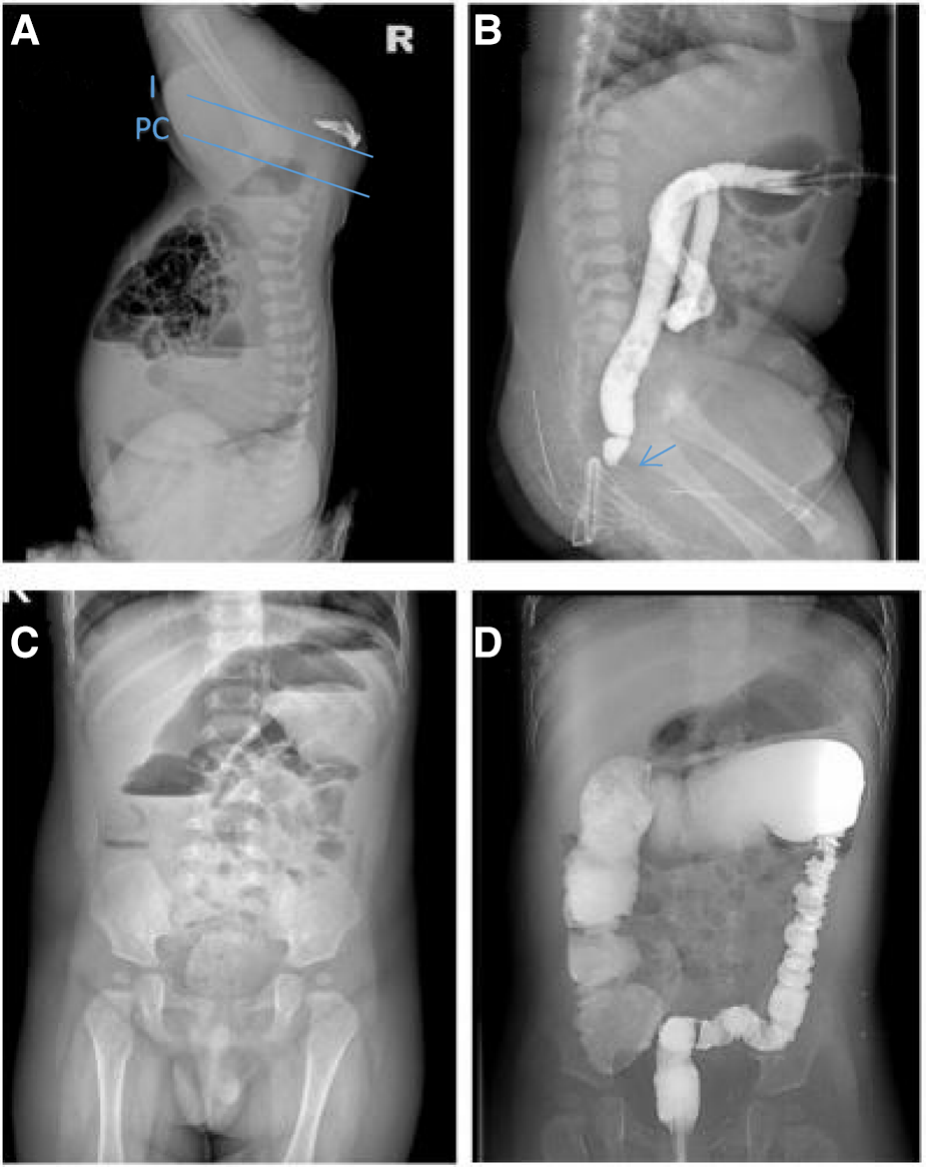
(A) abdominal x-rays before the first surgery. (B) before the second surgery. (C,D) after postoperative correction of anal atresia.
